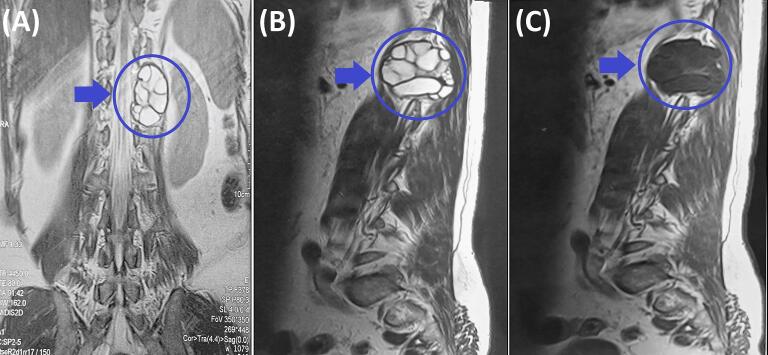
Preoperative thoracolumbar MRI. (A) Coronal T2-weighted image showing a multiloculated cystic lesion (blue arrow and circle) located paravertebrally in the thoracolumbar region from D11 to L1 invading T11-T12 and T12-L1 intervertebral foramen with limited invasion of the spinal canal. (B) Sagittal T1-weighted image demonstrating the cystic lesion's (blue arrow and circle) heterogeneous signal intensity and its proximity to the vertebral column. (C) Sagittal T2-weighted image further characterizing the multiloculated nature of the cystic lesion (blue arrow and circle).
